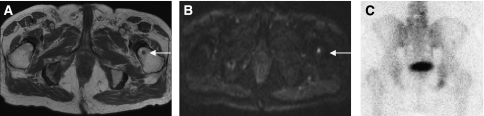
Comparison of MRI and MDP bone scintigraphy: pelvic T1W MRI (A) and b750 DWI (B) of a patient with carcinoma of the prostate shows a new small metastasis (arrows) involving the left neck of femur. This small intramedullary lesion has not evoked enough osteoblastic reaction to become visible on bone scintigraphy (C).
